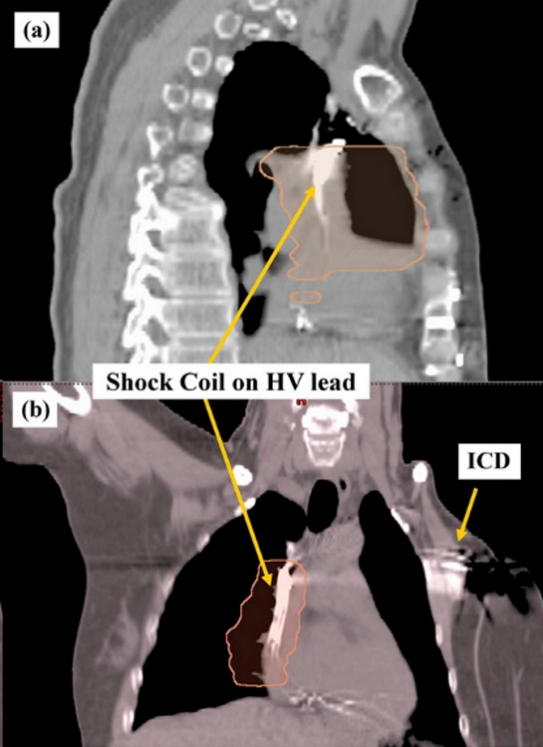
Sagittal (a) and coronal (b) computed tomographic images used in the treatment planning for a patient receiving proton radiotherapy for esophageal cancer. The patient's implanted cardioverter defibrillator (ICD) had a high‐voltage lead which had two pacing ring electrodes, a right ventricle shock coil, and a superior vena cava (SVC) shock coil, the last of which was located within the clinical treatment volume (CTV; pink shaded region).
